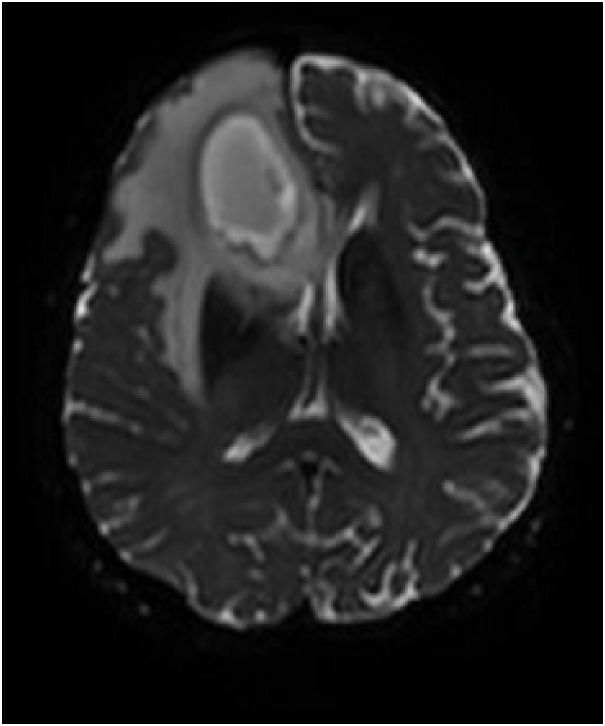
Axial section of the same lesion in diffusion-weightened imaging (DWI).
